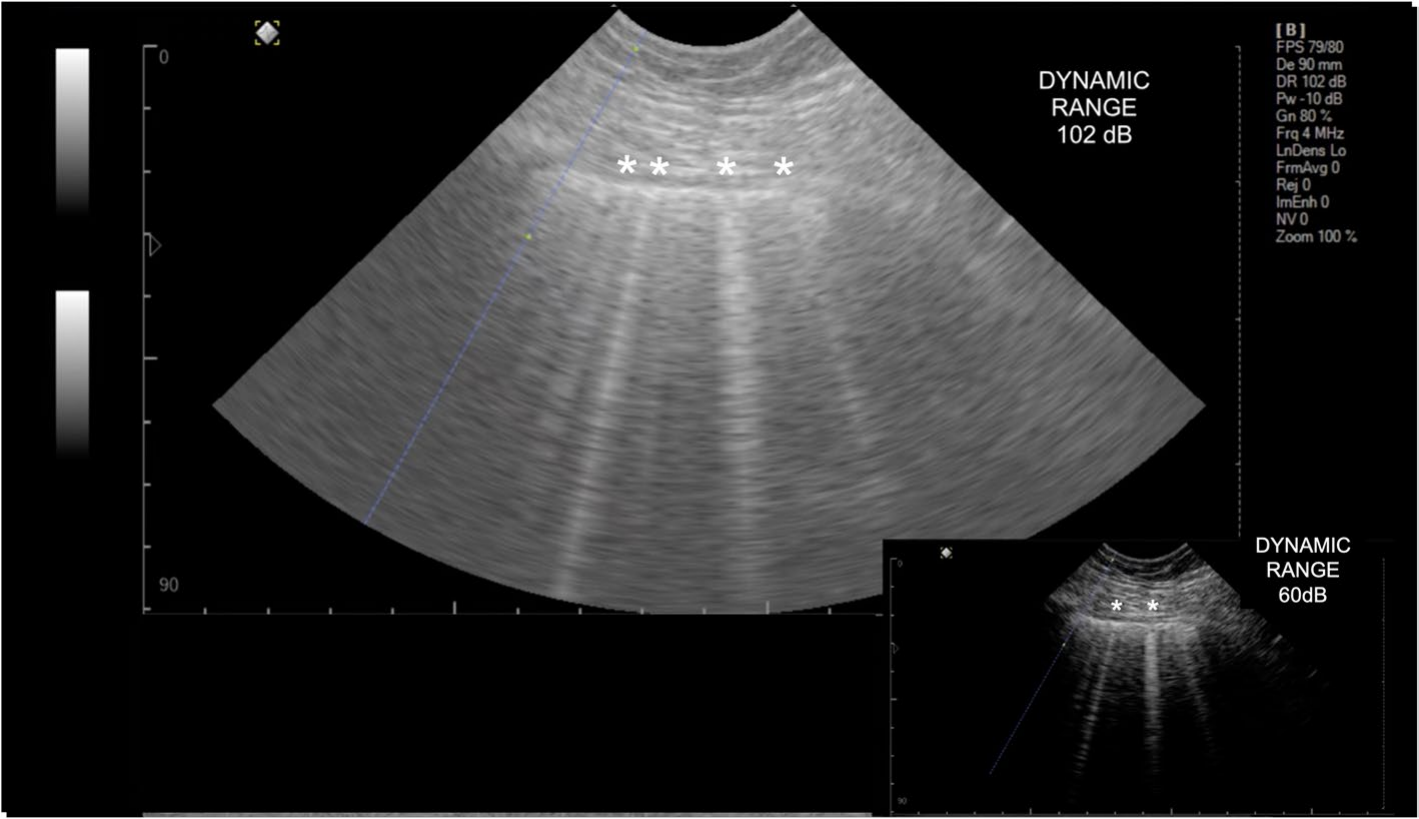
Influence of dynamic range on the number of B-lines identified (asterisk). In the bigger lung ultrasound image, more B-lines can be detected when using a dynamic range of 102 dB, compared to the smaller image at the inferior right corner, which was recorded using a dynamic range of 60 dB (four vs two B-lines)
